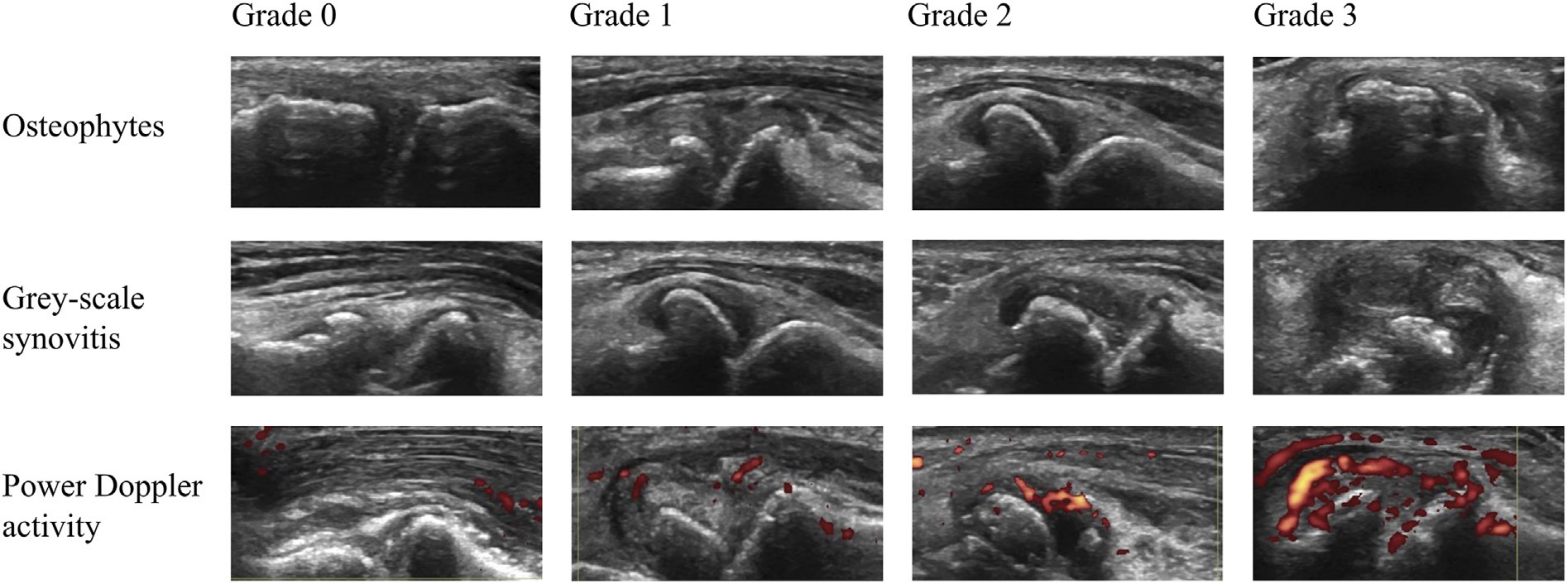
Example images from the ultrasound atlas of CMC-1 joints with grade 0 (no pathology), grade 1 (mild), grade 2 (moderate) and grade 3 (severe) pathology.
